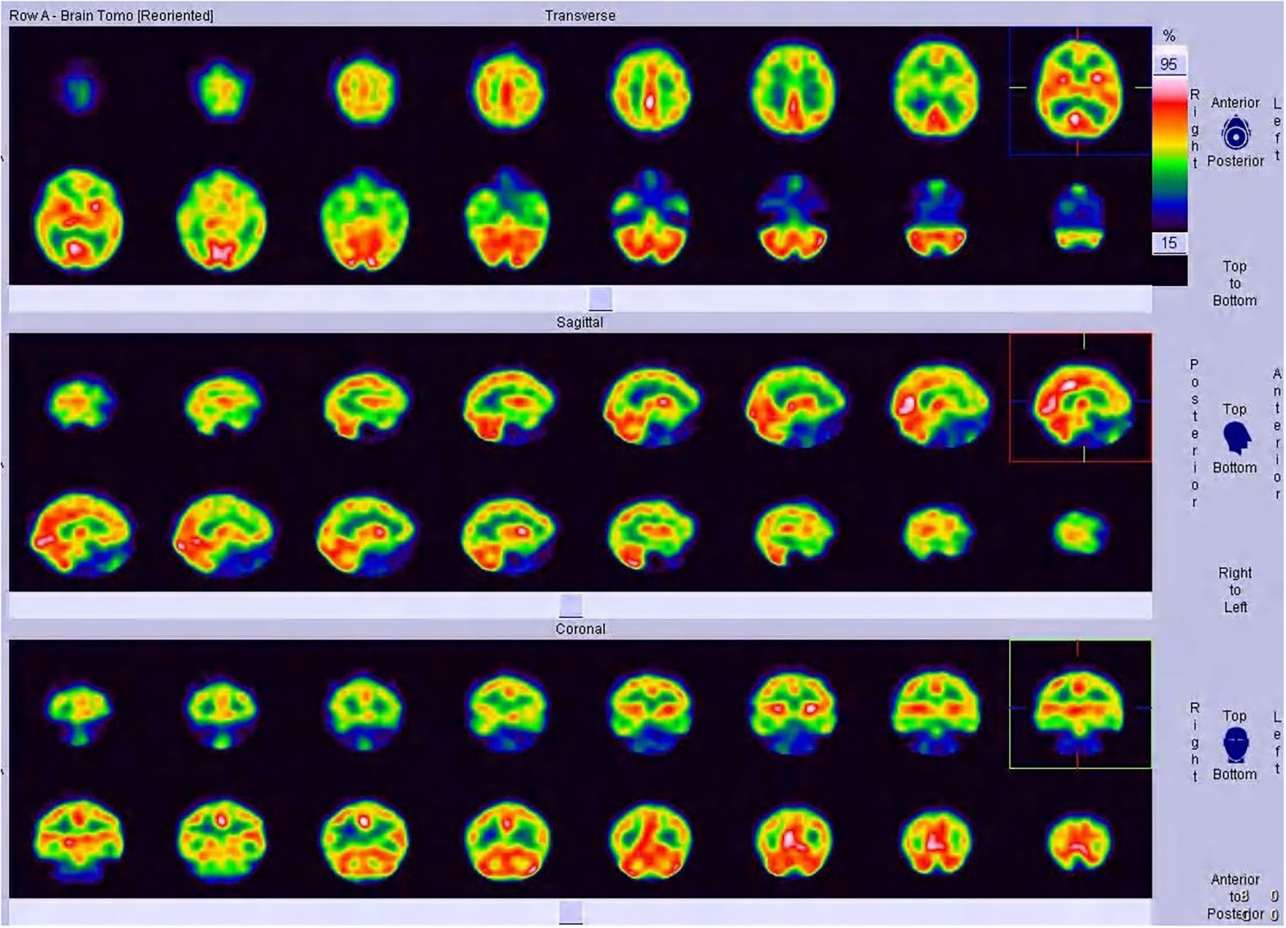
SPECT images of Case 2, a 19-year-old man.
